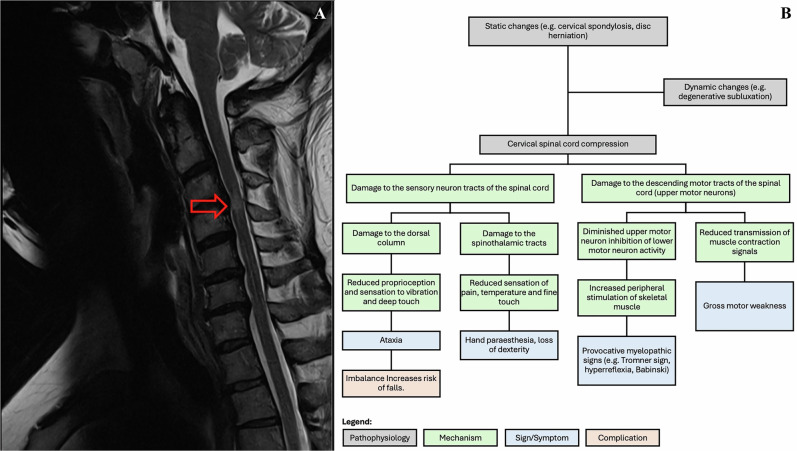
MRI Findings and Pathophysiological Overview of DCM. A T2-weighted sagittal MRI of a 45-year-old male with DCM, showing signal cord changes (indicated by red arrow). B An overview of the pathophysiological mechanisms, signs, symptoms, and complications associated with cervical spinal cord compression, including both static and dynamic changes.
